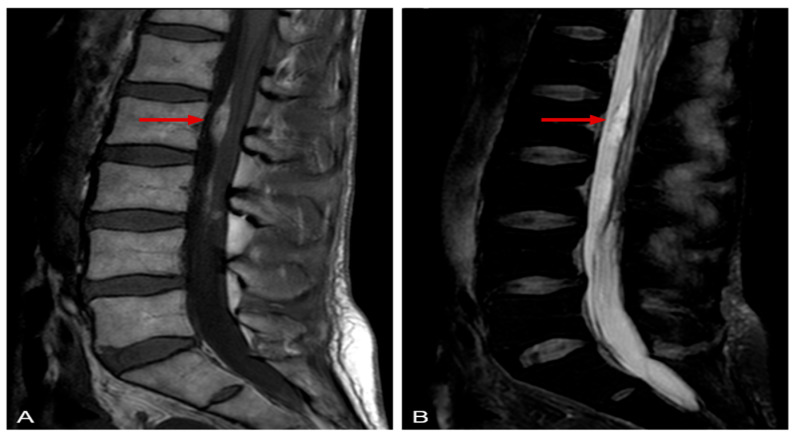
Lumbar spine magnetic resonance imaging (MRI). (A) T1-weighted sagittal image shows a band-like hyperintense lesion spanning the levels of lumbar vertebrae L1 to L3, without contrast administration. (B) T2-weighted fat-suppressed sagittal image demonstrates a similar band-like intradural hyperintense lesion, indicating the absence of fat content.
